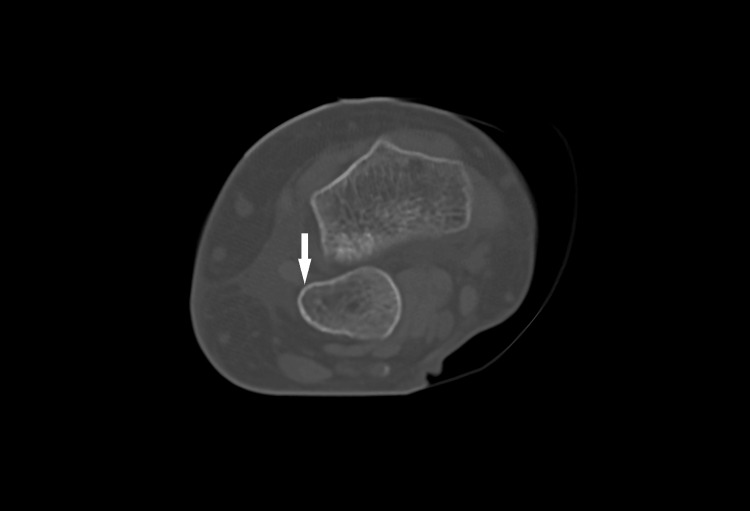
Axial plane CT scan of the right wrist showing volar (down) displacement of the ulna (white arrow) in relation to the radius. CT: computed tomography
